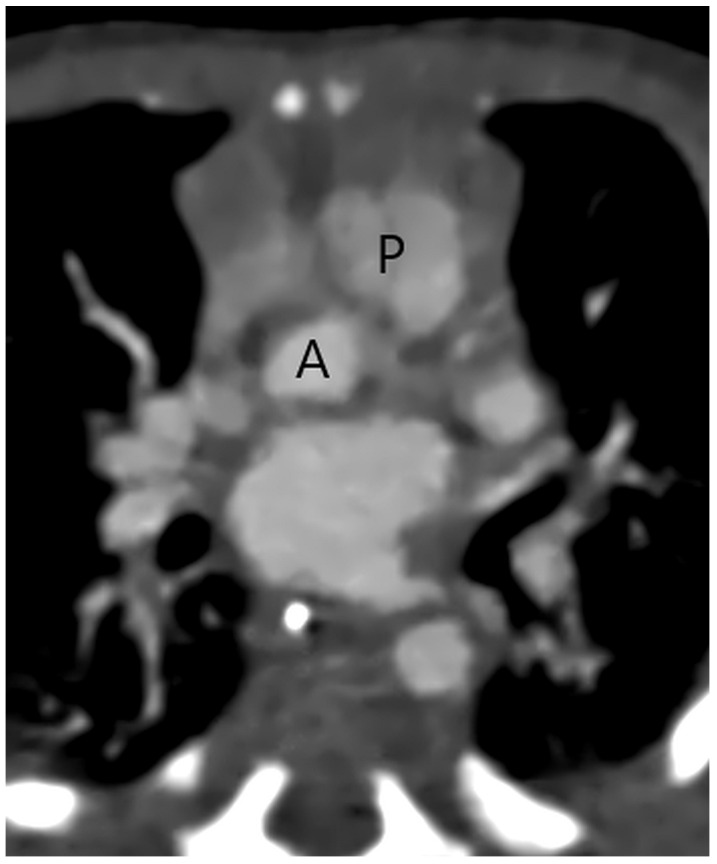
A separate aortic (A) and pulmonary (P) valve in a patient with a type 2 aortopulmonary septal defect at CT angiography.
